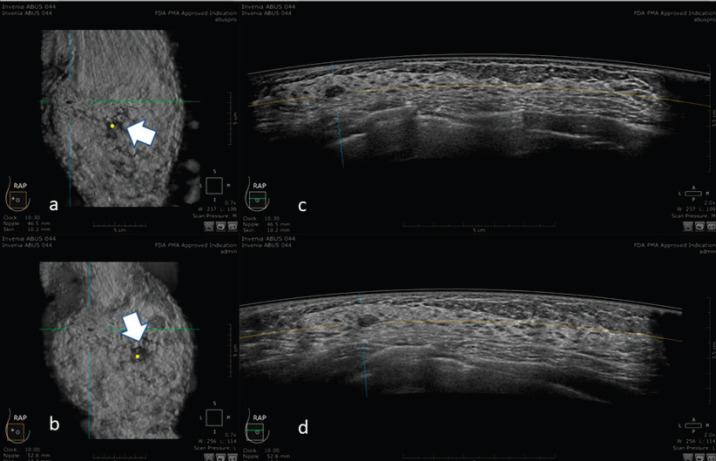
30-year-old patient with right breast carcinoma: a and b: 3D ultrasound (ABUS) image at the dedicated workstation. Reconstructed sagittal plane: the lesion is marked as a reference point. The nipple is marked with the arrow. c and d: 3D ultrasound (ABUS) image at the dedicated workstation. Reconstructed axial plane: the lesion is marked as a reference point.
